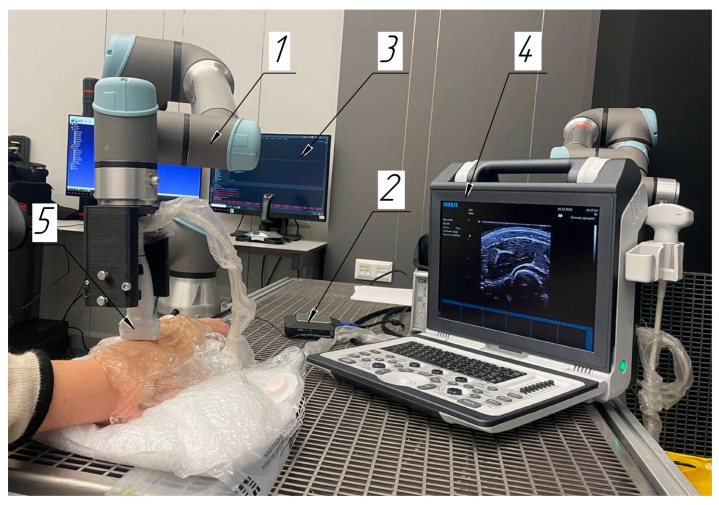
Components of the laboratory system: 1—collaborative 6-degree robotic manipulator; 2—video capture card; 3—personal computer; 4—digital ultrasound diagnostic system; 5—ultrasound sensor.
